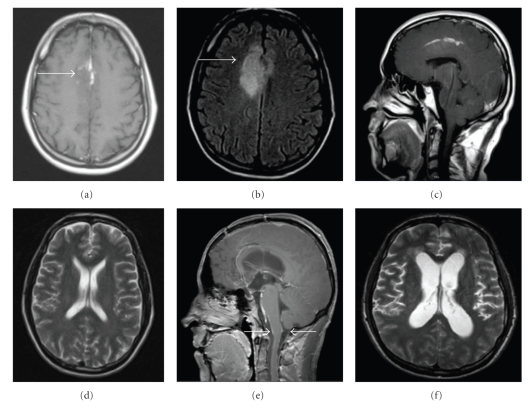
(a)–(d) Cranial MRI at initial presentation before surgical treatment of right frontal anaplastic oligodendroglioma. (a): axial T1-weighted contrast-enhanced MRI, (b) axial fluid-attenuated inversion recovery (FLAIR), and (c) sagittal T1-weighted contrast-enhanced MRI revealed a hyperintense lesion on the right cingulate gyrus with slight local mass effect (arrows). (d): T2-weighted axial MRI showing regular ventricular size. (e)-(f): MRI at second presentation 8 months after initial surgery. (e): sagittal T1-weighted contrast-enhanced MRI showing meningeal enhancement in the craniocervical junction (arrows). (f): Axial T2-weighted MRI showed marked ventricular enlargement.
